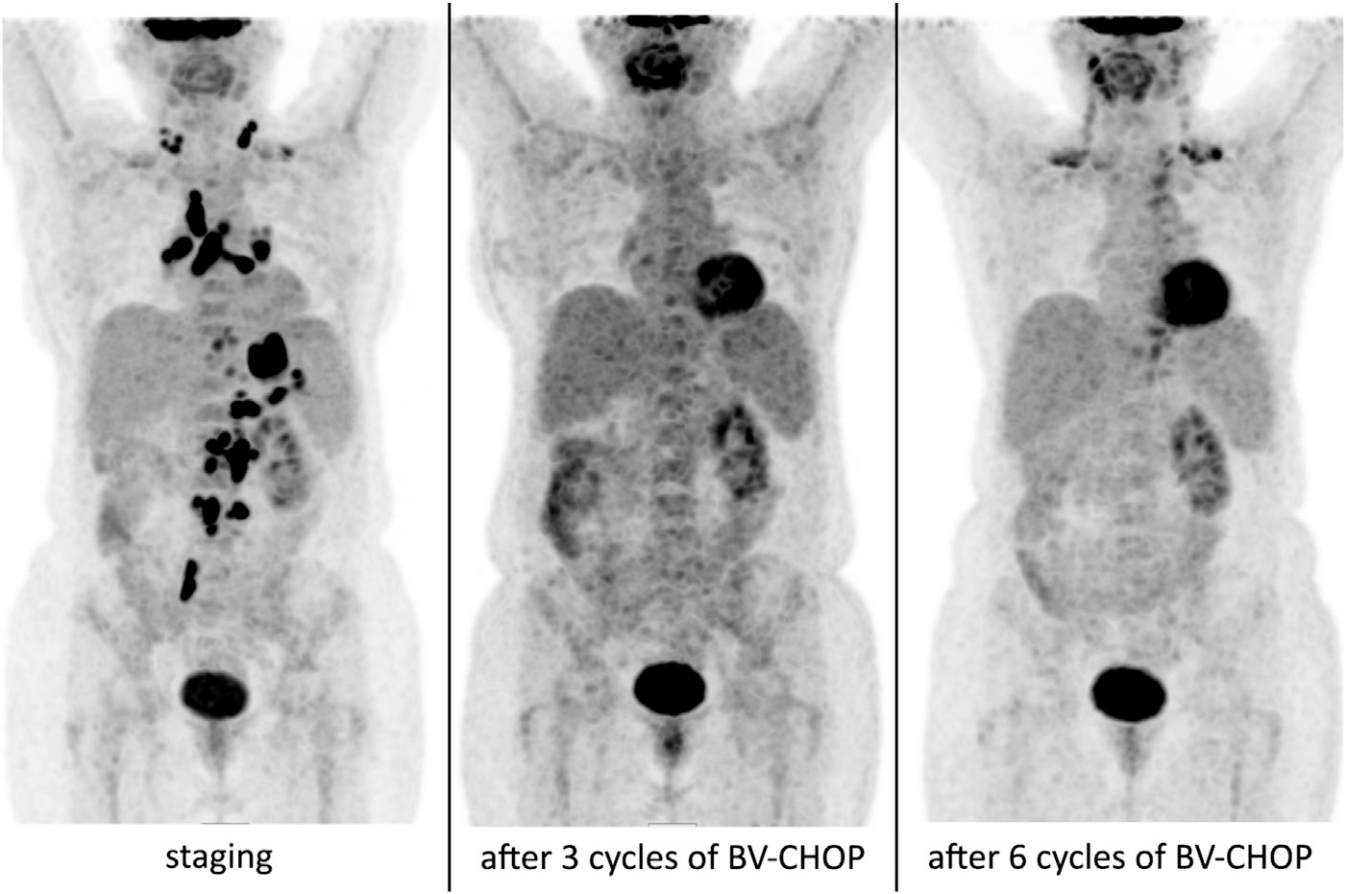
PET/CT scan results of our patient with grey-zone lymphoma, who received a regimen including brentuximab-vedotin + CHOP before, 3 cycles and 6 cycles after BV-CHOP therapy, respectively. The moderately enhanced FDG uptake on the post-induction (after six cycles of immunochemotherapy) image is due to brown fat activity. There is no clinical or radiological sign of relapse since the last PET/CT scan.
